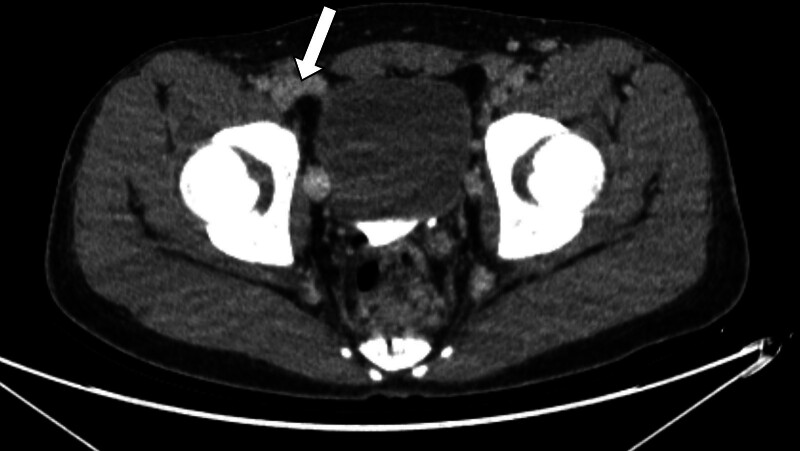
CT image of post-anticoagulation. Right external iliac vein thrombus resolution. CT = computed tomography.
